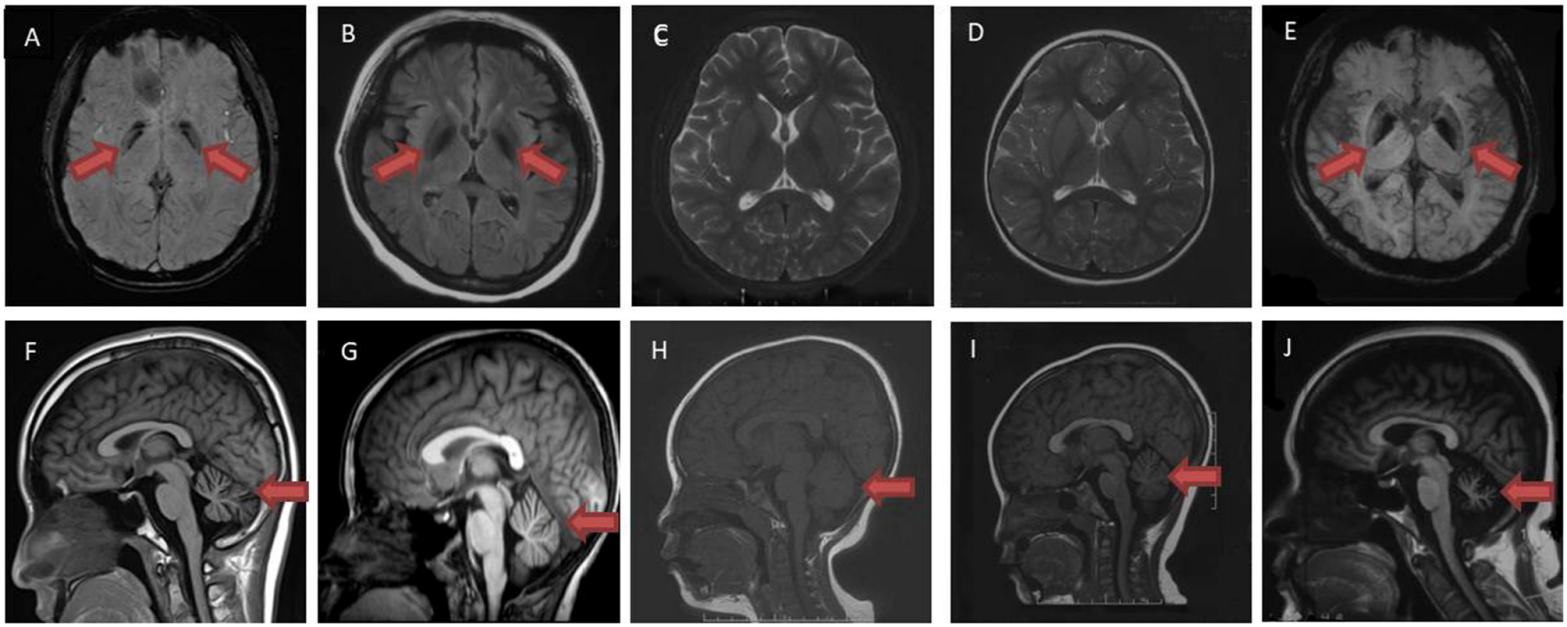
Brain magnetic resonance imaging examination of patient A II-1 (A,F), A II-2 (B,G), B-II 1 (C,H), B-II 2 (D,I) and C-II 1 (E,J). Susceptibility weighted imaging sequences (A,E) and T2 flair sequences (B) demonstrated iron deposition in bilateral globus pallidus (red arrow). No iron deposition was shown in T2 sequence (C,D) in patient B-II 1, B-II 2. T1 sequences demonstrated cerebellar atrophy in patient A II-1, A II-2, B-II 1, B-II 2, C-II 1 (F–J) (red arrow).
